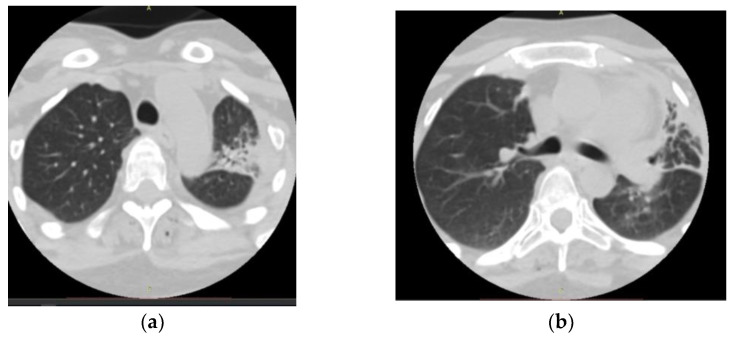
Chest computed tomography (CT) findings (February 2019): axial lung window setting showed atelectasis and a solid nodule surrounded by a halo of ground-glass attenuation in the left lung (superior segment and lingula), representing the “CT halo sign”. (a), axial section through trachea; (b), axial section below carina level.
